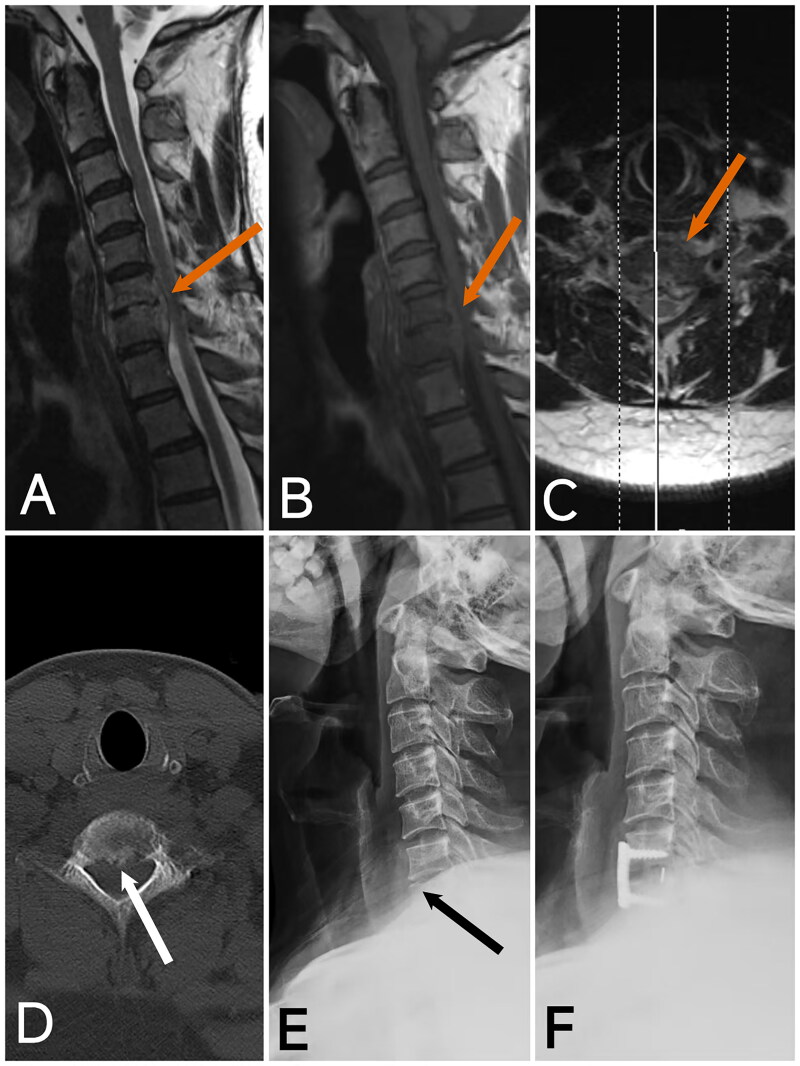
Image of case 5. A: T2-weighted imaging (T2WI); B: T1-weighted imaging (T1WI); C: Axial T2-weighted imaging (T2WI) shows an epidural abscess with significant compression of the spinal cord (red arrow). D: CT reveals prevertebral bone destruction (white arrow). E: Preoperative X-ray shows loss of intervertebral height at the C6-7 level (black arrow). F: Postoperative X-ray at 3 months demonstrates good positioning of the internal fixation, significant restoration of intervertebral height, and early fusion of the intervertebral bone graft.
